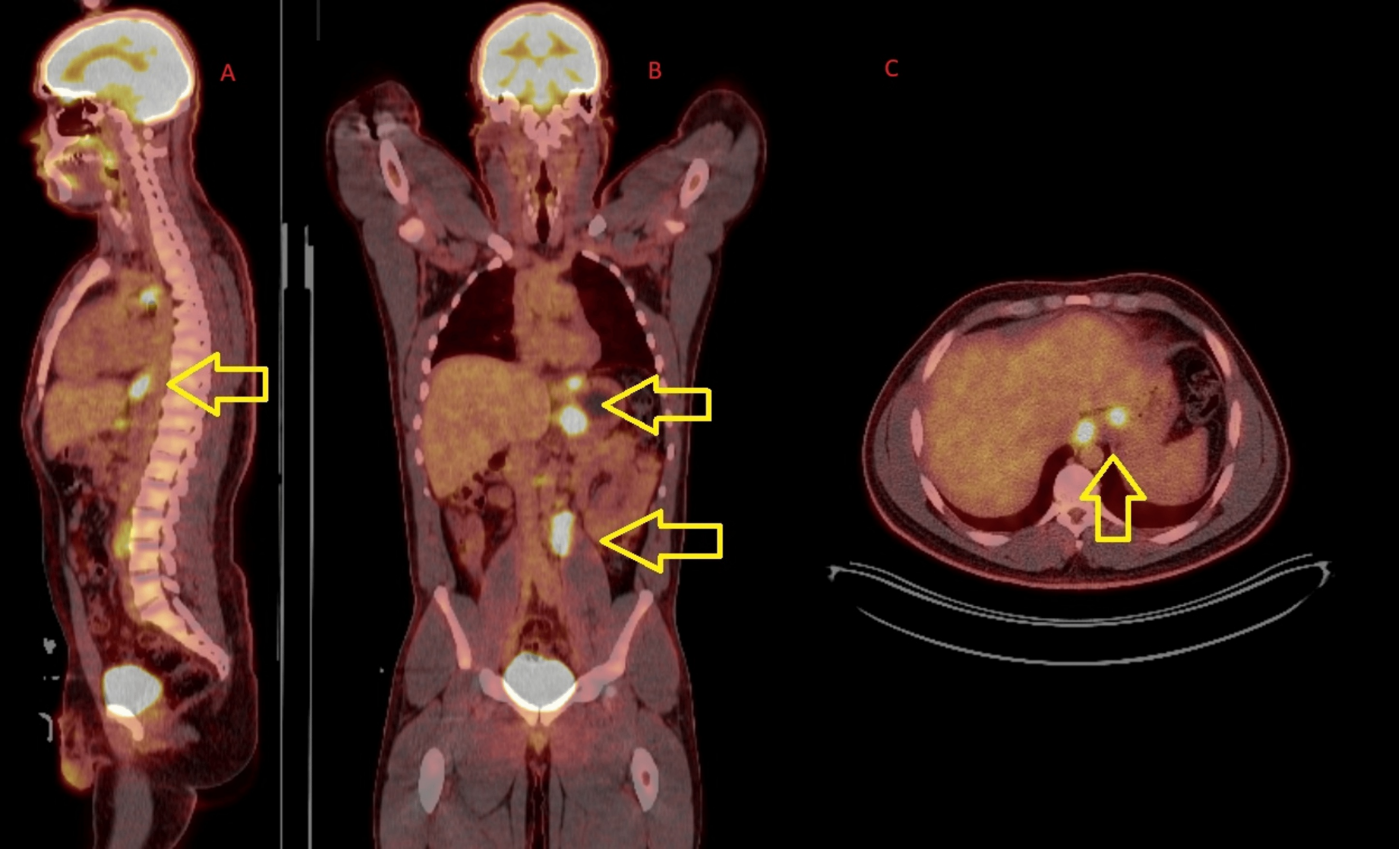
PET/CT scan showing a hypermetabolic distal esophageal adenocarcinoma primary with mediastinal, perigastric, and retroperitoneal lymph nodes The yellow arrows show the areas of hypermetabolic sites of cancer. (A) Sagittal view. (B) Coronal view. (C) Axial view.
